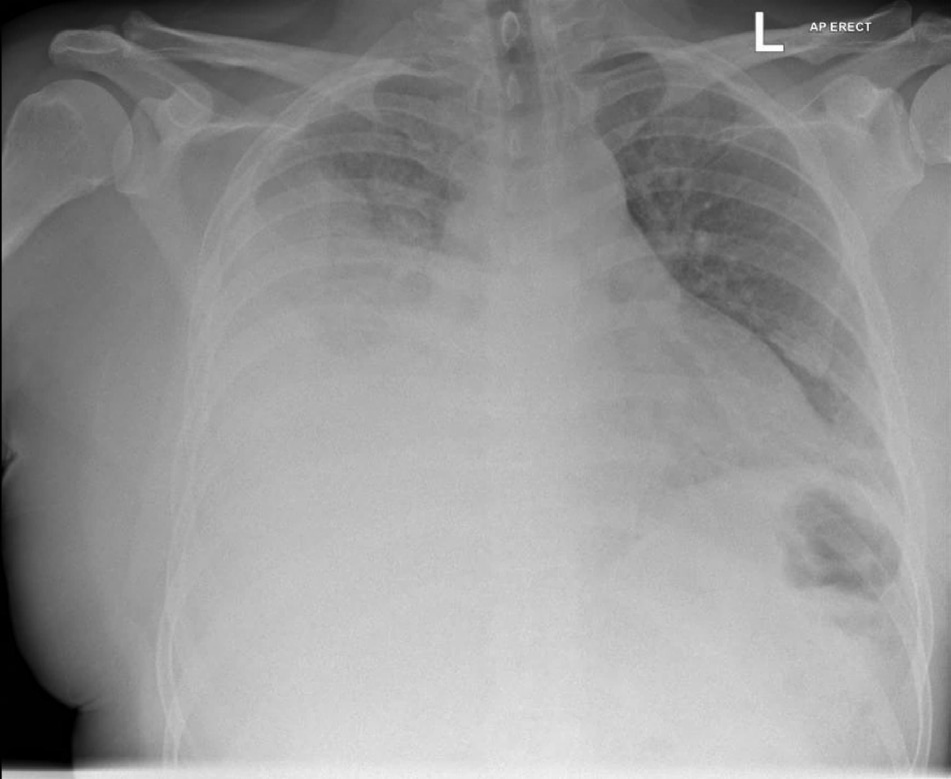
Chest radiograph on day of admission to hospital (day 4) showing right-sided opacification
